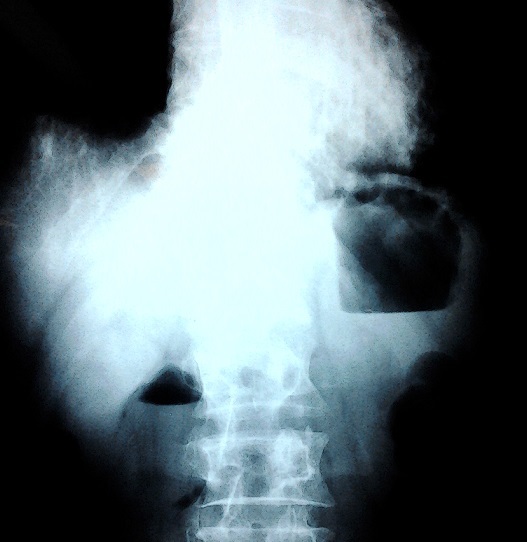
Radiographie de l'abdomen : notez la superposition des deux niveaux hydro- aériques, le grand traduisant la stase gastrique, le plus petit l'obstruction duodénale
